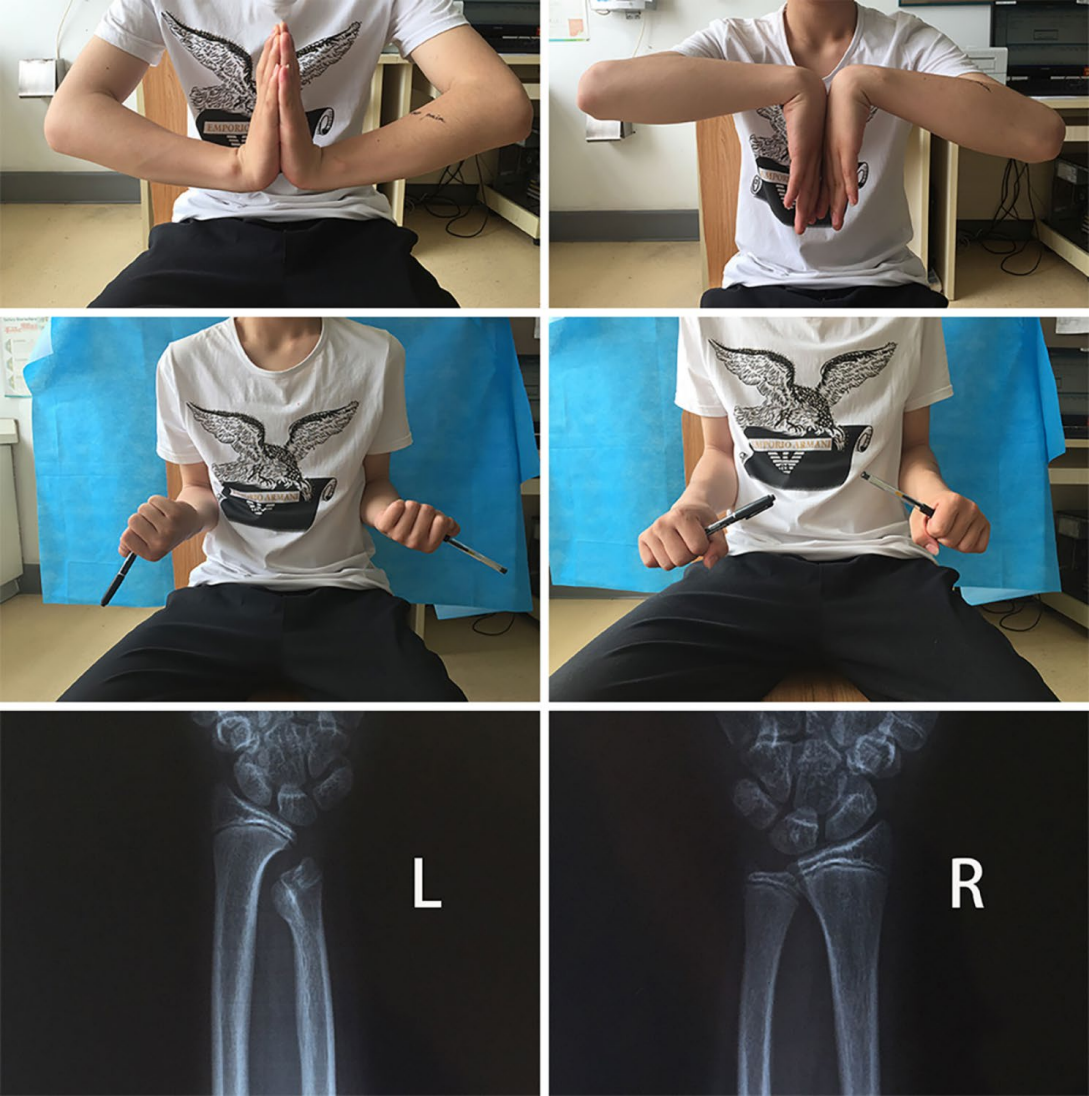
Movement and X-rays at 3 years of follow-up
